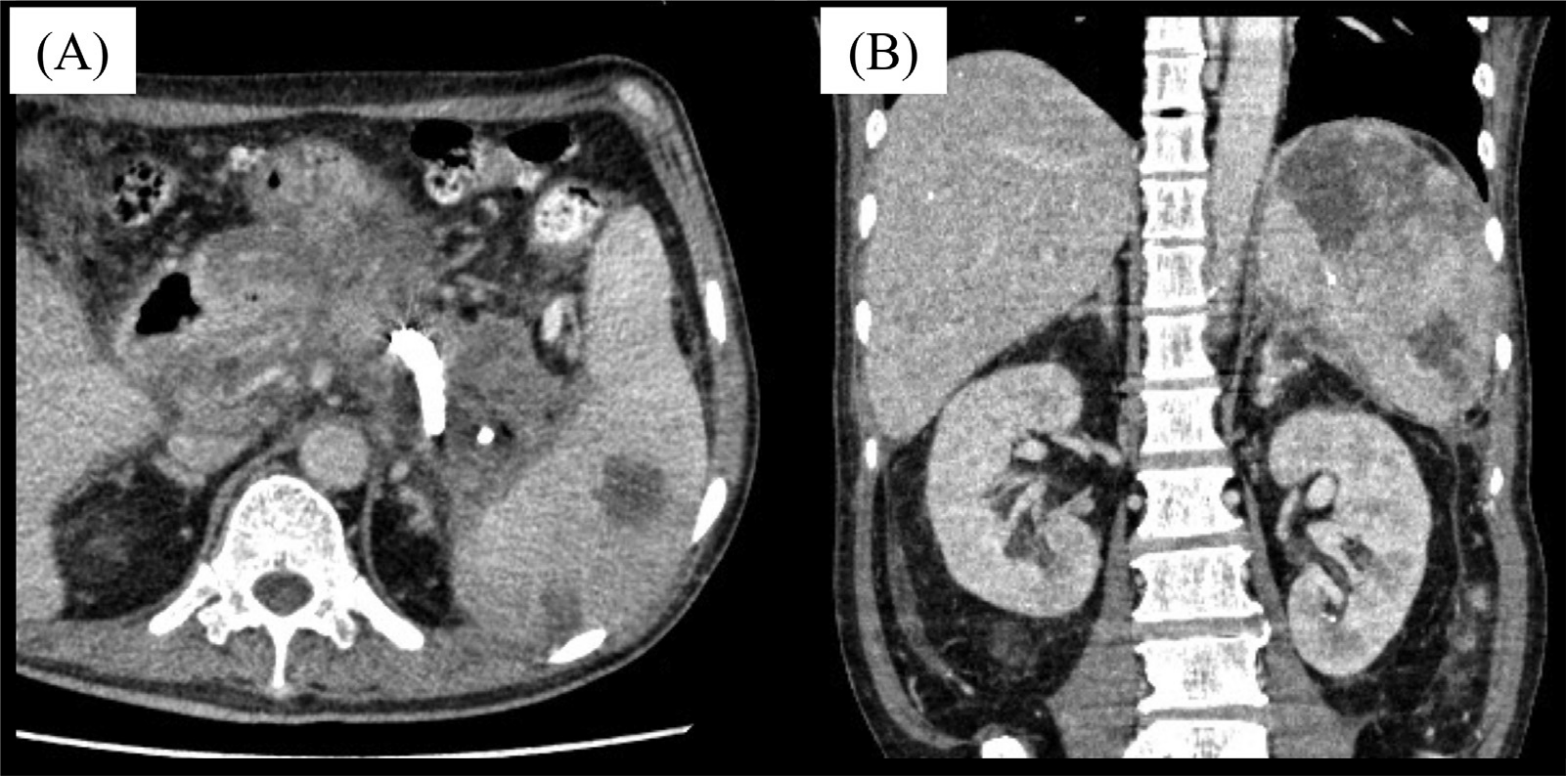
Contrast-enhanced CT after DEN treatment. (A) The WON around the pancreas has been reduced in size. (B) Embolic material remains in the splenic artery. Partial splenic infarction is seen.
